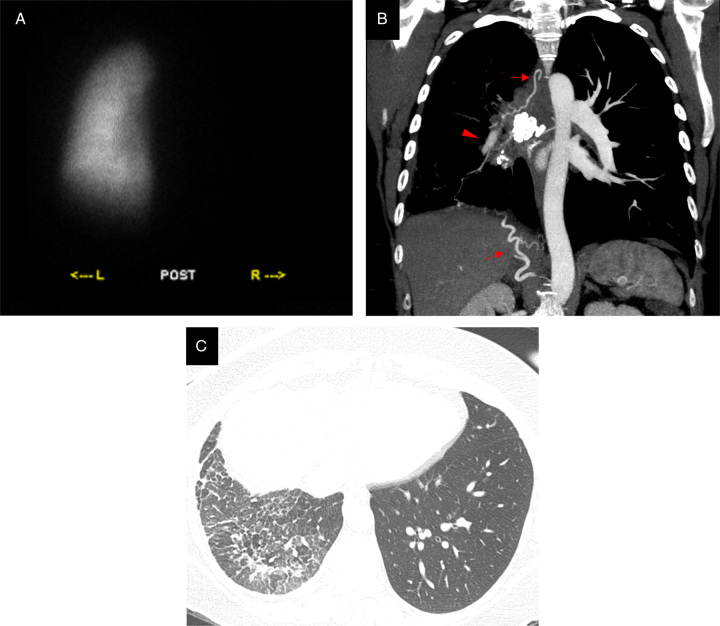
A 42-year-old woman with fibrosing mediastinitis. A, The perfusion scan shows no visible perfusion in the right lung, with quantified perfusion of 2% in the right lung, compared with 98% in the left one. Dynamic ventilatory images (not shown) demonstrated mildly decreased ventilation in the right lung on a single-breath image, which appeared to equilibrate throughout the washout phase. B, Coronal maximum intensity projection reconstruction of contrast-enhanced CT shows a mostly calcified mass in the right hilum compressing the ipsilateral pulmonary artery, which appears of reduced caliber and hypoattenuating (arrowhead). Hypertrophied collateral vessels can be recognized (arrows). C, Lung windows show unilateral right-sided irregular septal thickening and reticular abnormality, with bronchovascular distortion indicating fibrosis.
